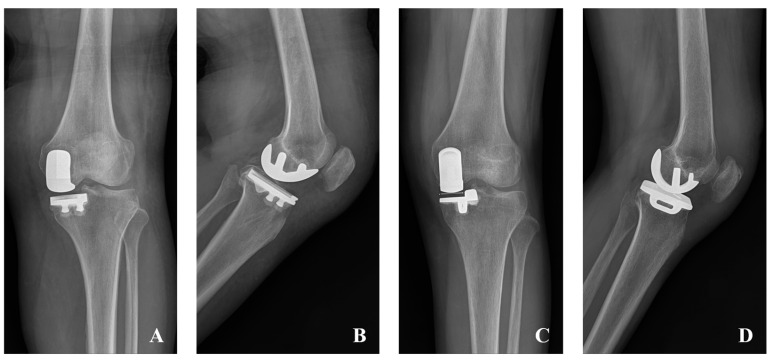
Postoperative anteroposterior (AP) (A) and lateral (B) radiographs of fixed-bearing medial unicompartmental knee arthroplasty (UKA). Postoperative AP (C) and lateral (D) radiographs of mobile-bearing medial UKA.
